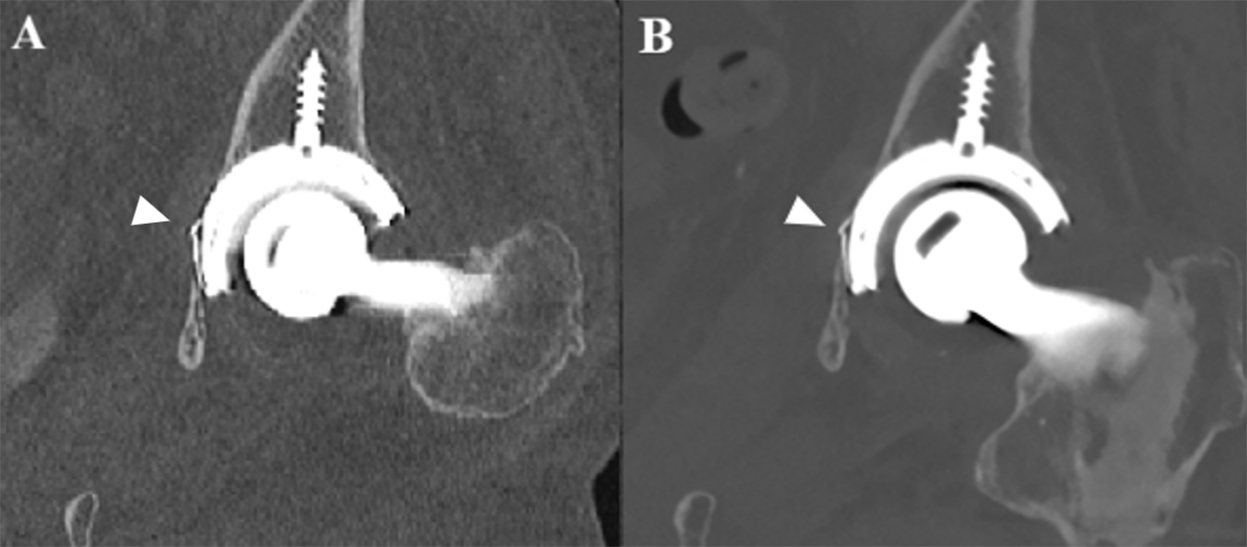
An 81-year-old female consulting the emergency department after trauma with an implant fracture of the acetabular cup of the hip prosthesis. (A) Coronal slice with IMAR showing an implant fracture (white arrowhead) on a Siemens SOMATOM Definition Flash. (B) An additional IMAR PCCT scan was performed on the Siemens NEAOTOM Alpha to enhance characterization of the implant fracture (white arrowhead) highlighting the improved spatial resolution and metal artifact reduction at a lower radiation dose compared to EID CT (CTDIvol of 11.8 mGy vs 13.3 mGy).
